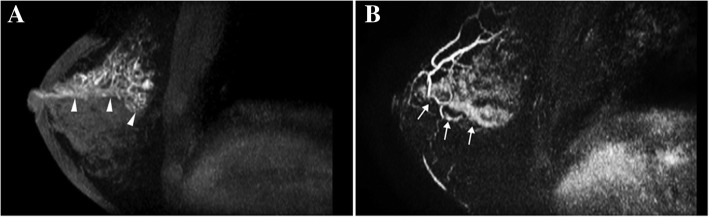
A case of papilloma with DCIS manifesting as segmental enhancement (in 53-year-old woman). a The MIP of the pre-contrast T1-weighted image reveals duct dilation (arrowheads) in the right breast. b The MIP of the subtracted image shows abnormal enhanced lesion with segmental distribution (arrows). The pathology is intraductal papilloma with DCIS
